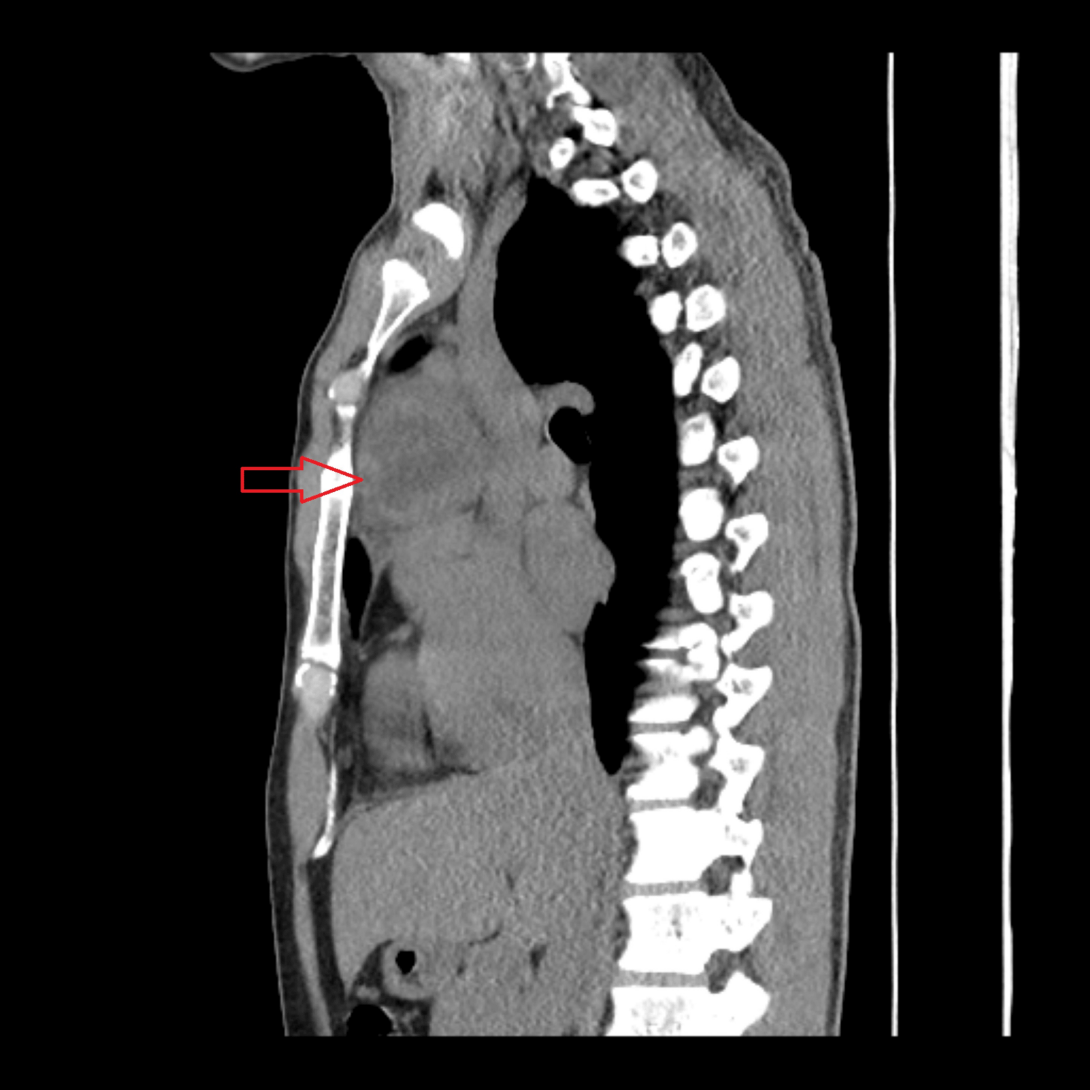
Sagittal CT scan image exhibiting an anterior mediastinal mass and its relationship with surrounding structures
